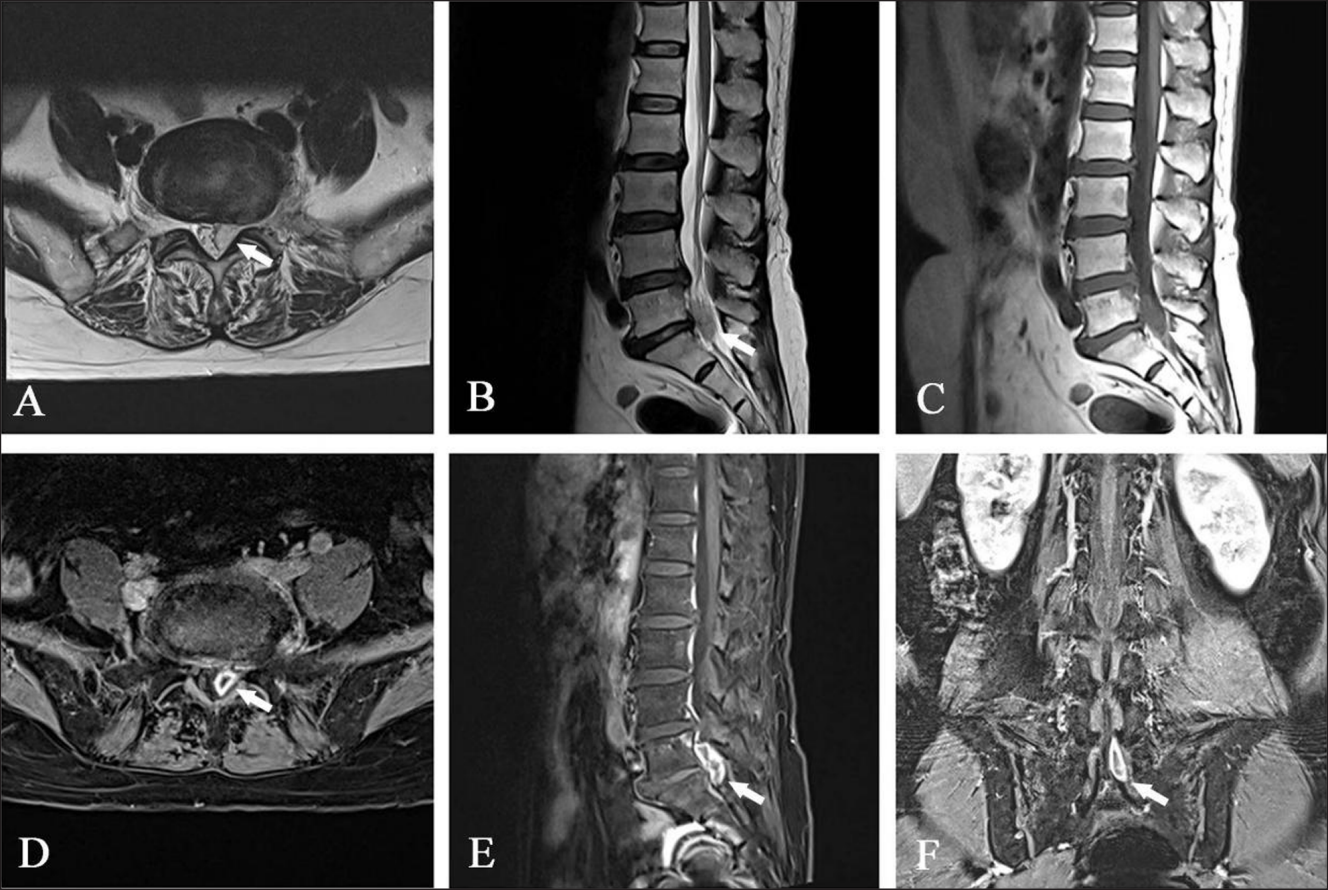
Postoperative: Axial T2-weighted MR image without contrast (A) shows a hyperintense nodule (arrow) in the spinal canal; sagittal image without contrast shows that the nodule (arrow) is hyperintense on T2WI (B) and isointense signal on T1WI (C); fat-suppressed T1-weighted axial (D), sagittal (E) and coronal (F) MR images show the nodule (arrow) displays a “ring” enhancement after gadolinium administration.
